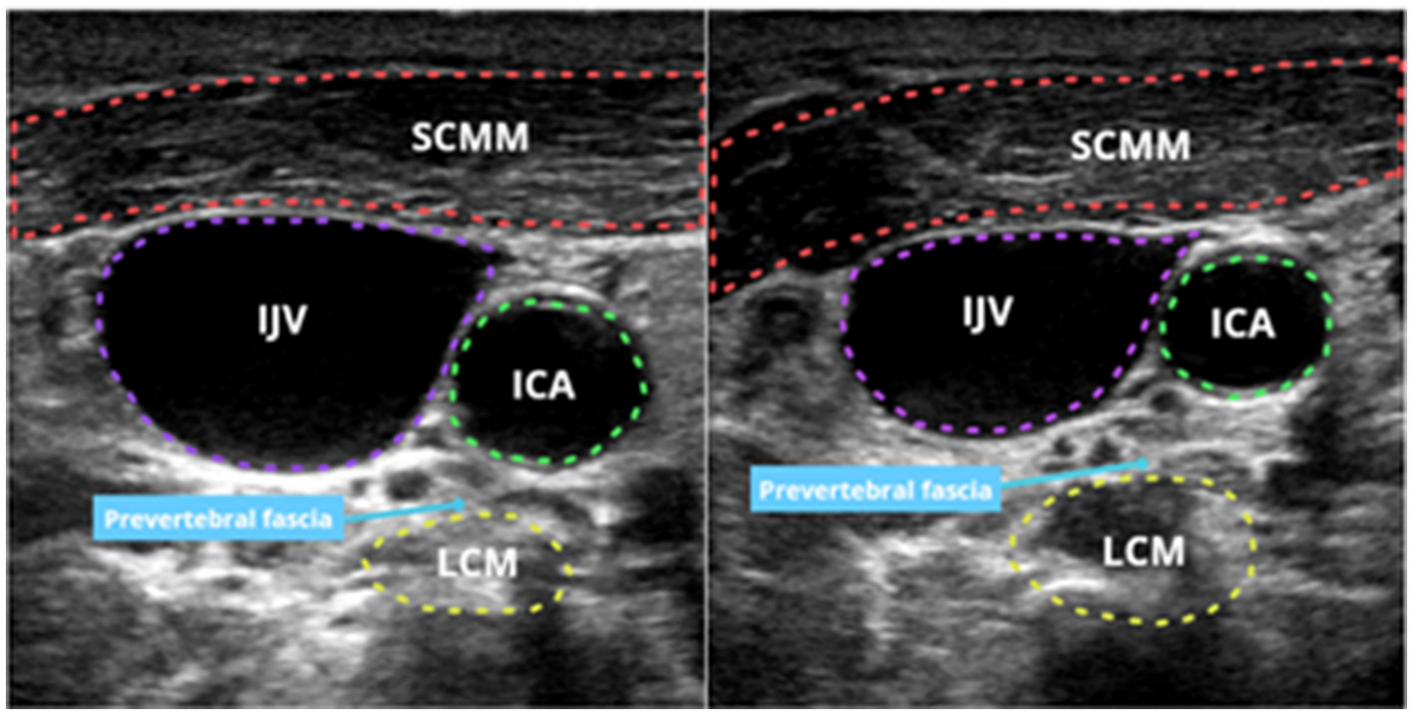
Ultrasound-guided stellate ganglion block before and after.
